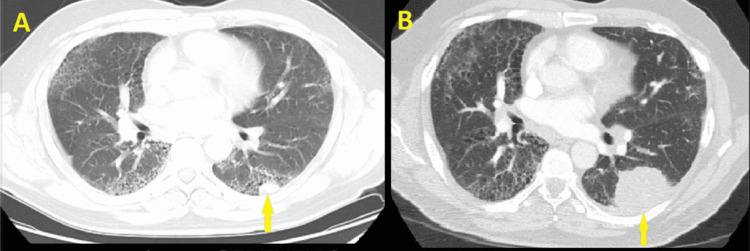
Computed tomography without contrast of the chest (A) and computed tomography angiography of the chest (B) (A) Computed tomography (CT) without contrast shows a mass-like area of consolidation seen toward the superior segment of the left lower lobe measuring 1.3 cm x 1.9 cm x 2.9 cm (yellow arrow). There is diffuse honeycomb changing in the bilateral lower lobes, right middle lobe, and peripherally along the upper lobes. There is subpleural consolidation in the upper lobes bilaterally to the apices, and no endobronchial lesion or central bronchiectasis. (B) CT angiography of the chest shows an interval growth of the previously reported left lower lobe nodule, presently measuring 7.1 cm x 3.9 cm, compatible with malignancy (yellow arrow). There is mediastinal and bilateral hilar lymphadenopathy, left greater than right. Positive emphysematous and fibrotic changes involve pulmonary parenchyma. There is no evidence of pneumothorax, central occlusive pulmonary embolism (PE), pulmonary arterial hypertension, right heart strain, pericardial effusion, or thoracic aortic dissection or aneurysm.
